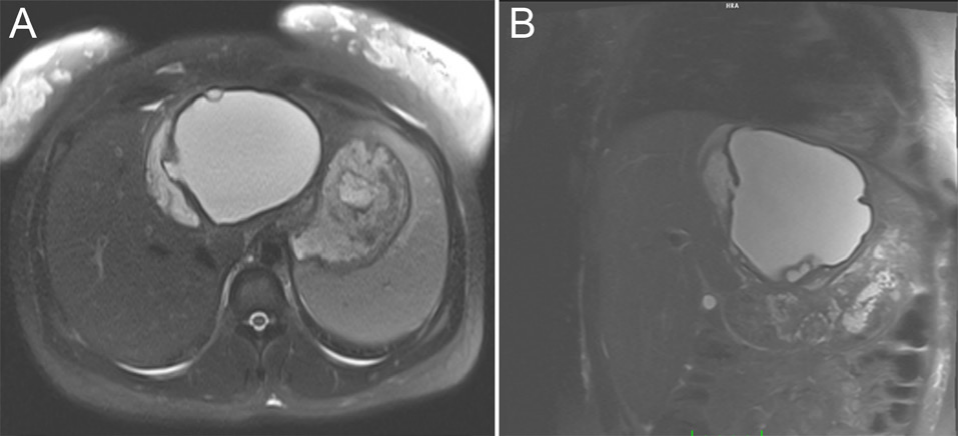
Axial (A) and coronal (B) T2-weighted magnetic resonance image shows intrahepatic rupture of the left lobe hydatid cyst of the liver.
